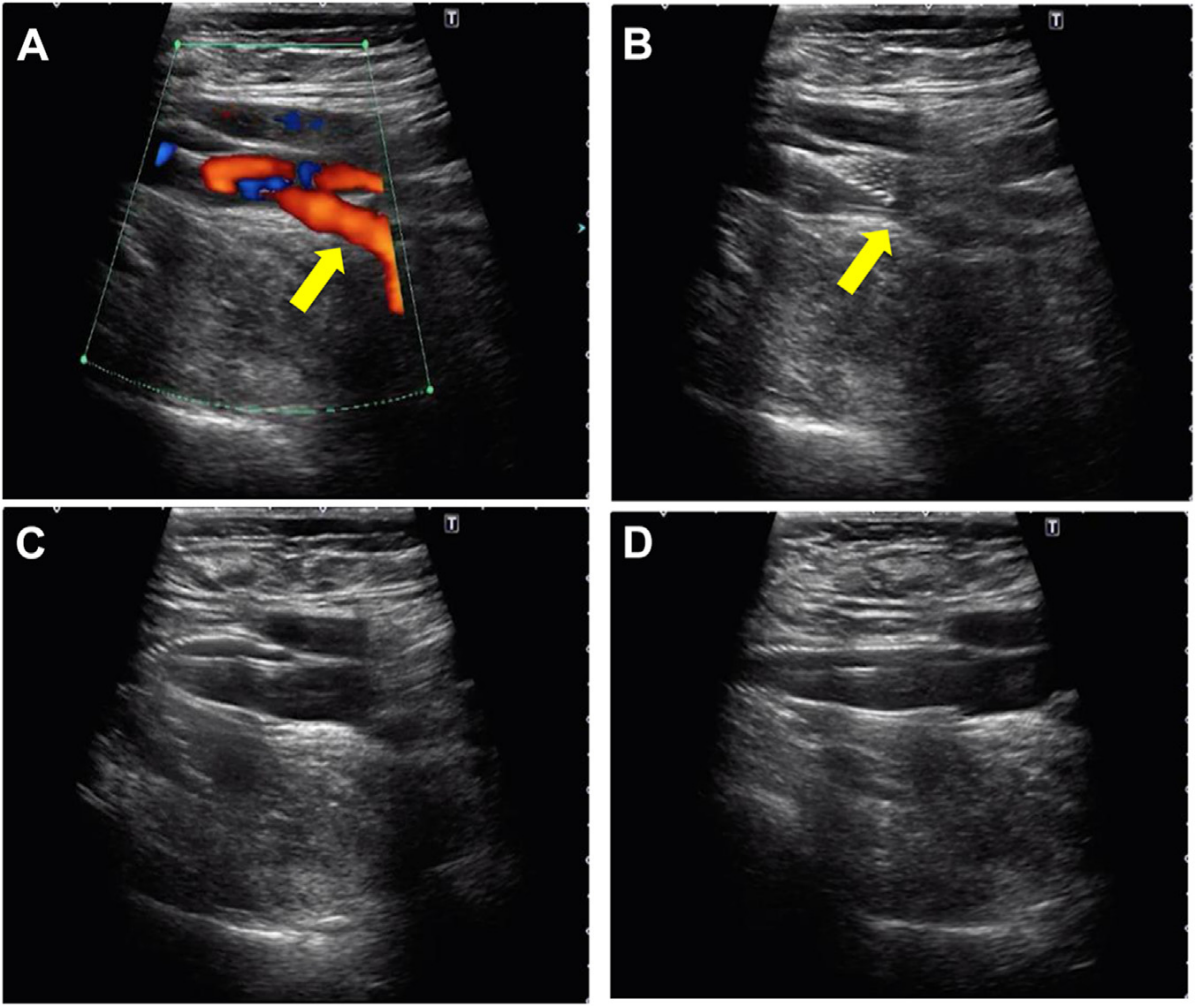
Ultrasound-guided stenting in the common femoral vein (CFV) just above the deep femoral vein (DFV) confluence. A, Color Doppler ultrasound showing blood flow from the DFV (*arrow*). B, The distal end of the stent was positioned just above the DFV confluence (*arrow*) under ultrasound guidance. C,D, The stent was deployed with careful adjustment of the distal end.
